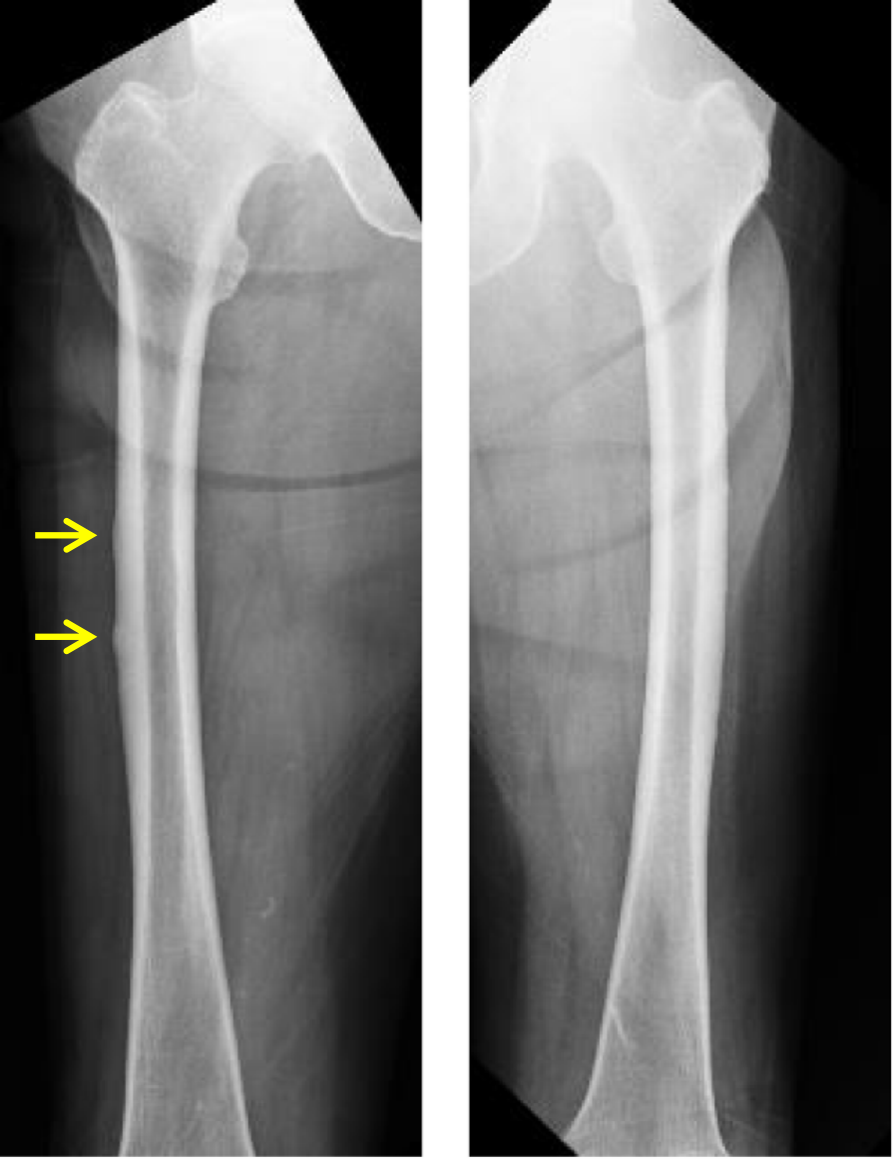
Radiography of the femur. The two yellow arrows indicate external bone reaction
